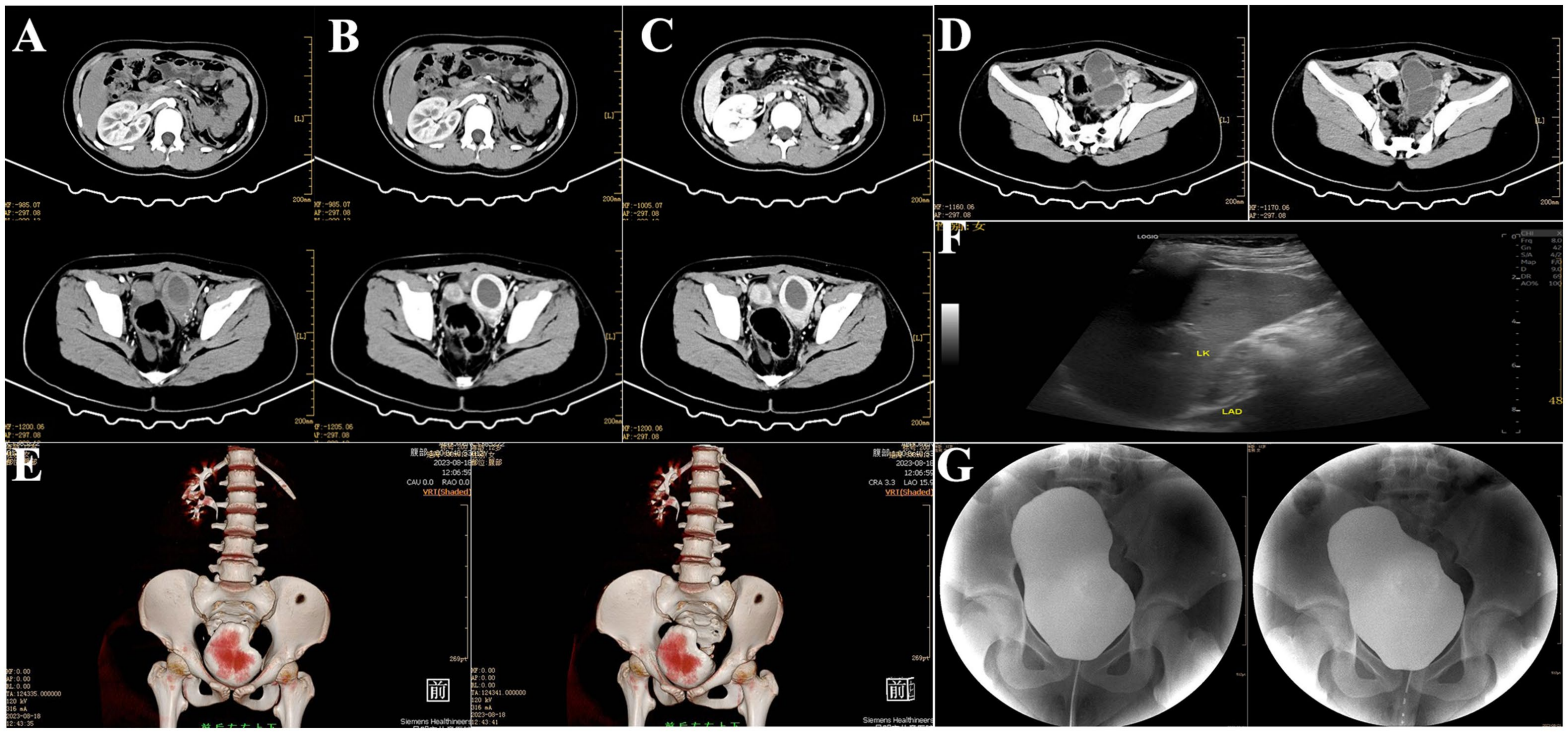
Preoperative imaging findings. (A–C) Contrast-enhanced cross-sectional CT of the abdomen and pelvis revealed bilateral uterine malformations with a left-sided hematoma. (D) Enhanced CT image of the left pelvic mesothelial cyst. (E) Three-dimensional reconstruction of abdominal and pelvic CT. (F) Preoperative urinary system and gynecological ultrasound. (G) Preoperative retrograde urography.
